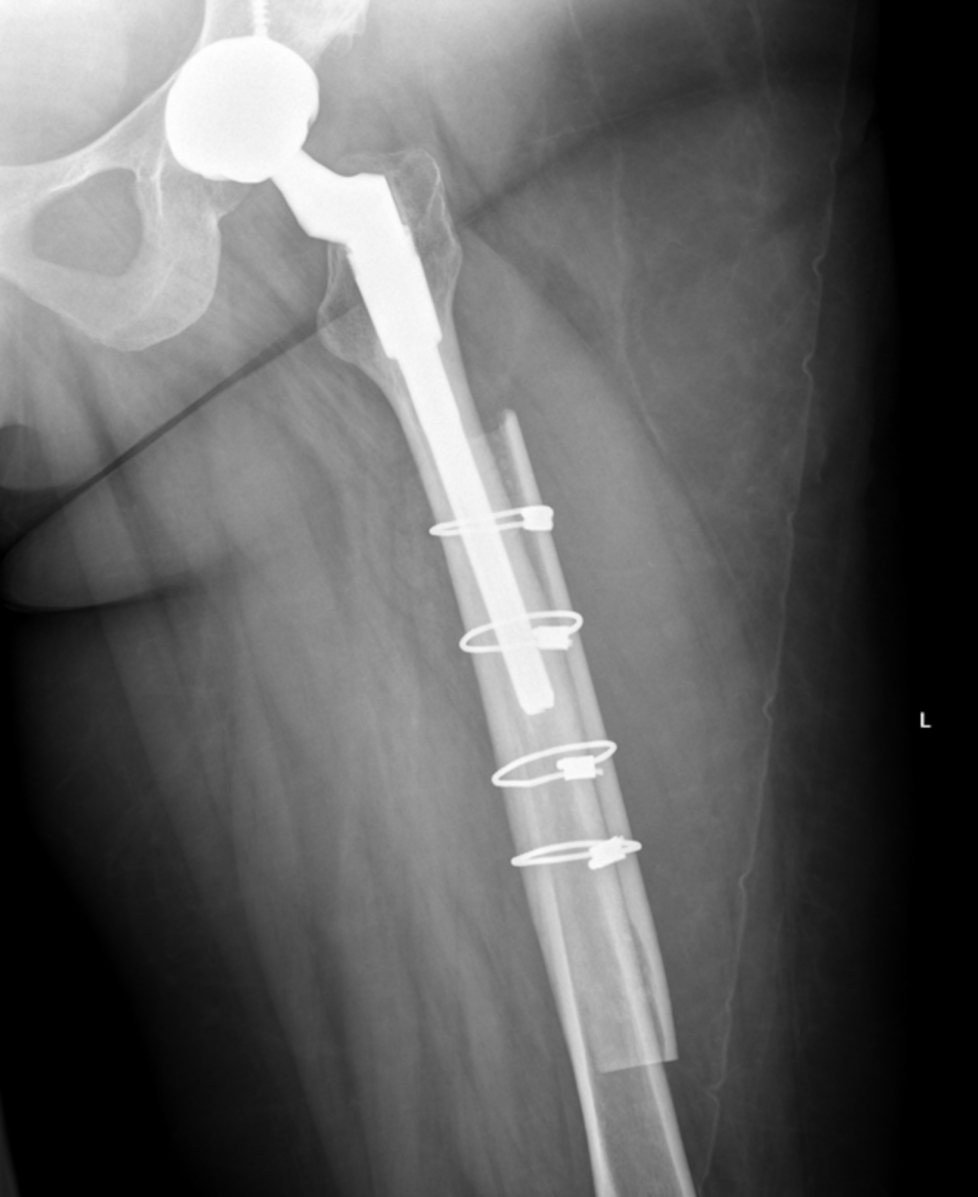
AP radiograph one day post-operatively of the left femur cortical strut allograft. AP, anteroposterior
